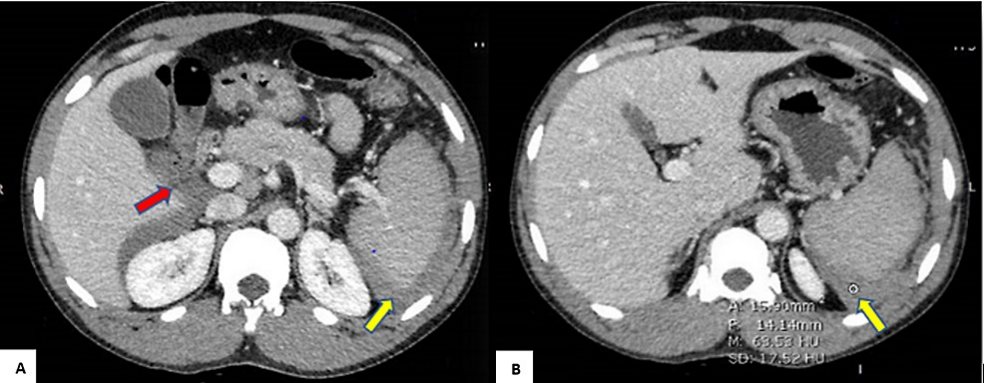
A) & B) computed tomography with intravenous contrast, axial cuts showing right upper abdomen hemoperitoneum (red arrow), and perisplenic hematoma (yellow arrows)
